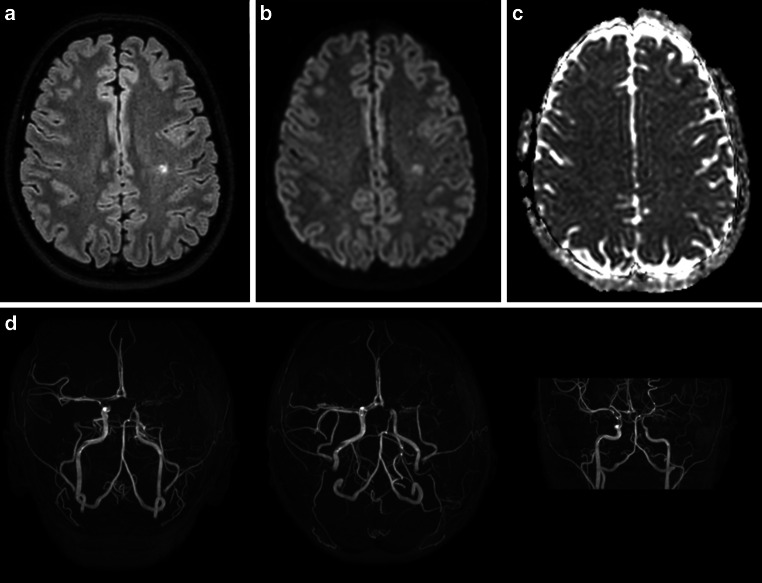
Fluid-attenuated inversion recovery (FLAIR; a) combined with diffusion-weighted imaging (b) and corresponding apparent diffusion coefficient map displayed in (c) revealed a subacute postischemic lesion in the left-hemispheric white matter. Time-of-flight (TOF) magnetic resonance angiography (MRA; d) showed multiple changes of arterial vascular calibers (with considerably reduced flow signals of the bilateral internal carotid artery [ICA] and its proximal branches)
